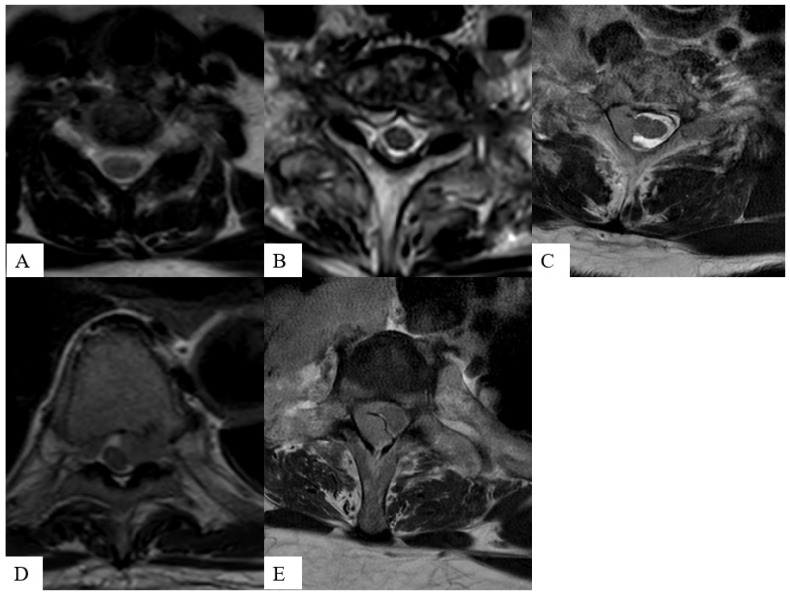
Axial T2-weighted images demonstrate the increasing severity of the epidural space involvement in patients with spinal metastasis from images (A) to (E): ESCC grade 1a shows tumor spreading to epidural space without indenting the thecal sac (A); grade 1b shows tumor causing indentation of the thecal sac (B); grade 1c shows tumor abutting but not compressing the spinal cord (C); grade 2 shows tumor compressing the spinal cord (D); and grade 3 shows tumor completely obliterating the subarachnoid space (E).
